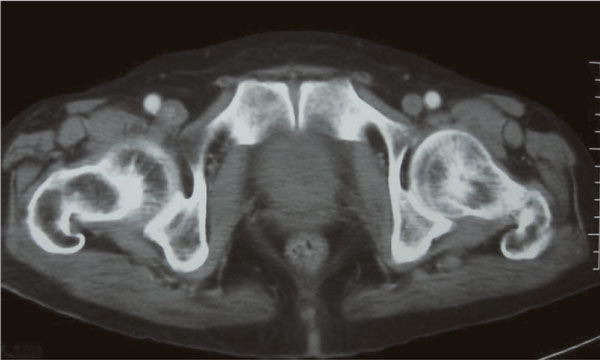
CT scan of the pelvis performed after chemotherapy shows complete radiological response of the prostatic tumour.
